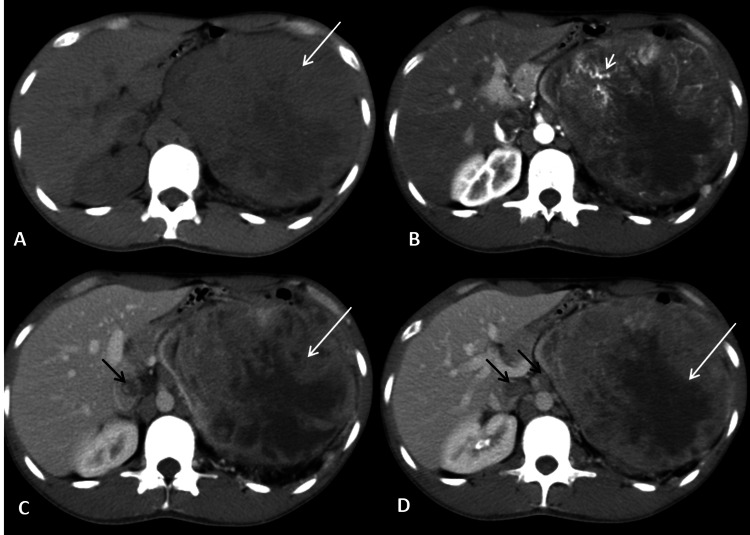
Axial NCCT image revealed a large heterogeneous mass in the left renal fossa (A, long arrow), showing neovascularization on arterial phase images (B, short arrow). The mass demonstrated heterogeneous post-contrast enhancement with non-enhancing necrotic areas within on the venous phase (C, D, long arrow). Heterogeneous enhancing tumor thrombosis was seen in the IVC and left renal vein (C, D, black arrows). NCCT: non-contrast computed tomography; IVC: inferior vena cava
